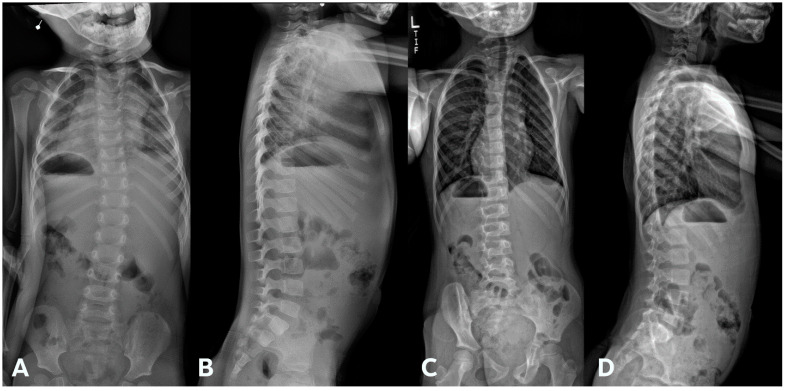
(A,B) Initial PA and lateral radiograph of a 2-year-old child presenting with 17° of scoliosis at the cervicothoracic junction and T1 slope of 11°. (C,D) Follow-up radiographs showing a stable curve approximating 18° 4 years later.
